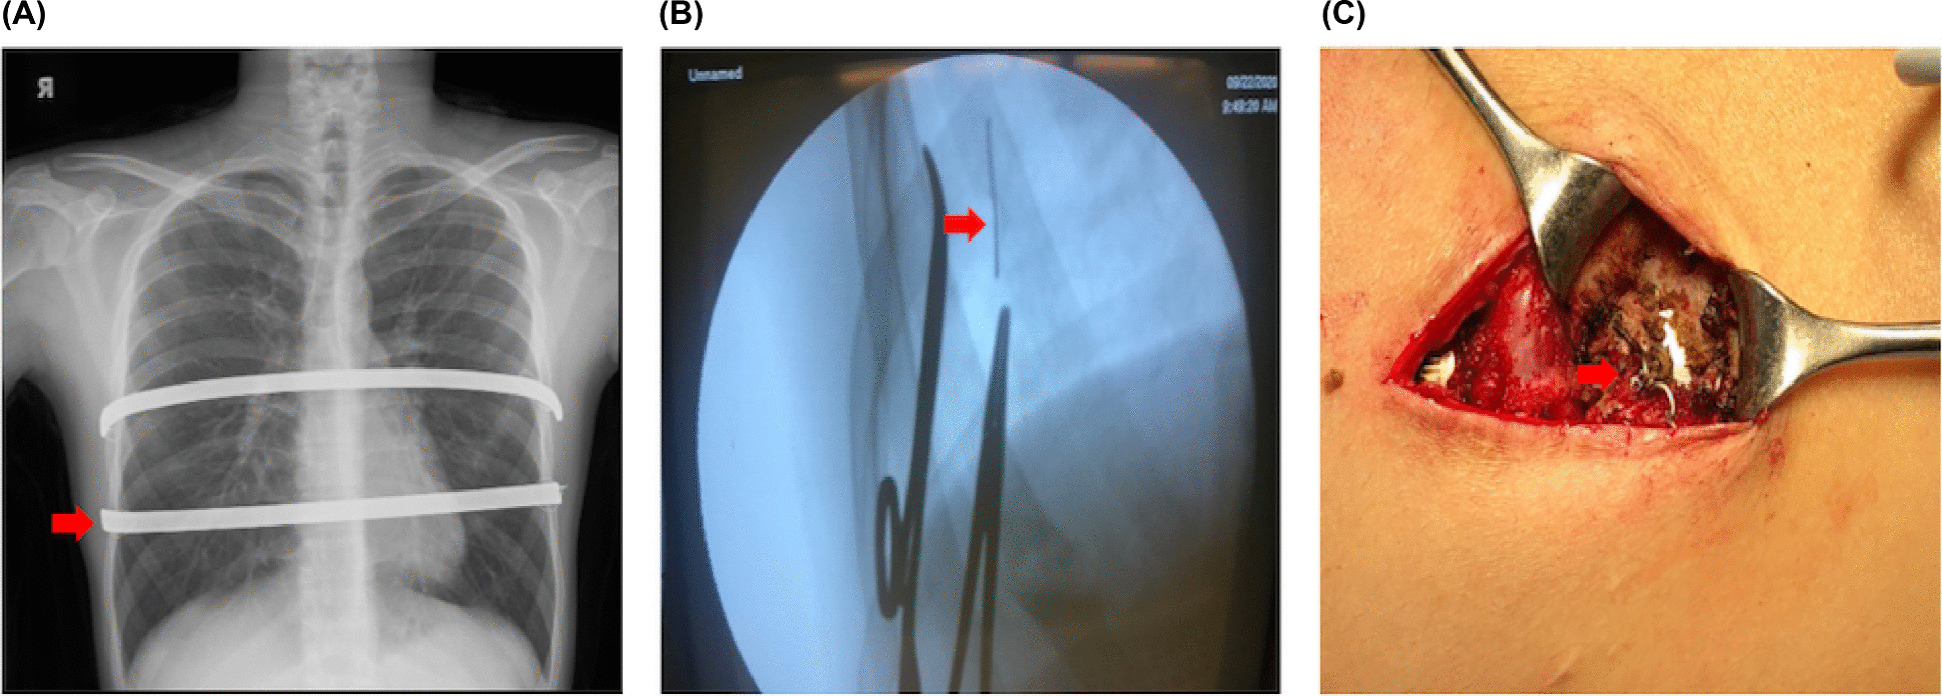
Radiologic and perioperative features.  Radiologic and perioperative features with bar removal 5 years post-correction. A  Segmental wire fracture on the right of the lower pectus bar (red arrow). B  Wire fragment (red arrow). C  Callus formation encasing wire (red arrow). CXR, chest radiograph
